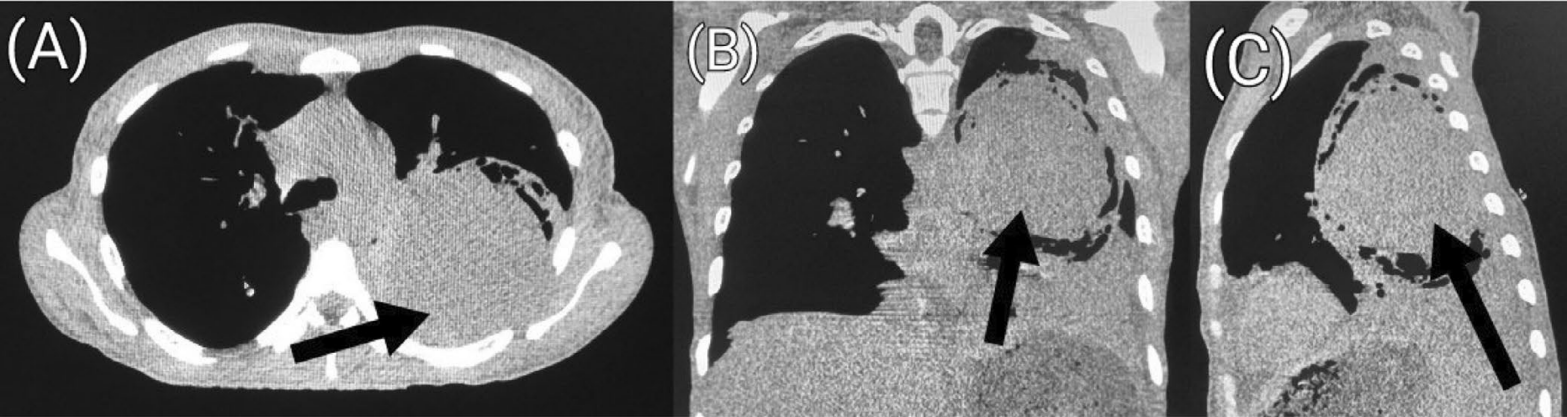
Pre‐contrast axial (A), coronal (B), and sagittal (C) CT scan images of the chest in soft tissue window demonstrating a large cavity (black arrow) in the upper lobe of the left lung.
